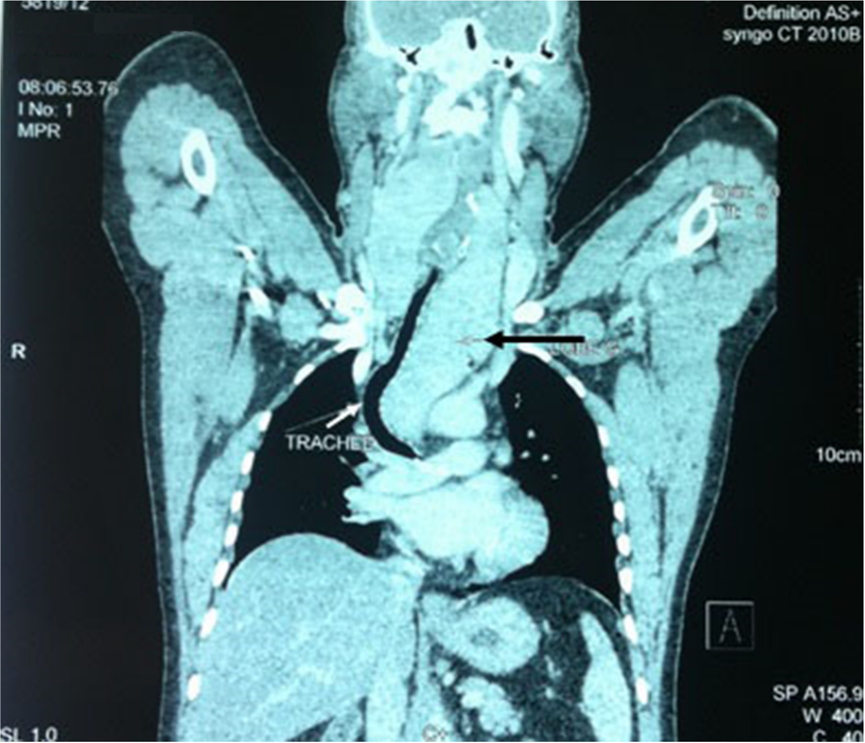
Coronal CT scan showing a giant goiter extending into the mediastinum (black arrow), laminating and deflecting the trachea to the right (white arrow).
